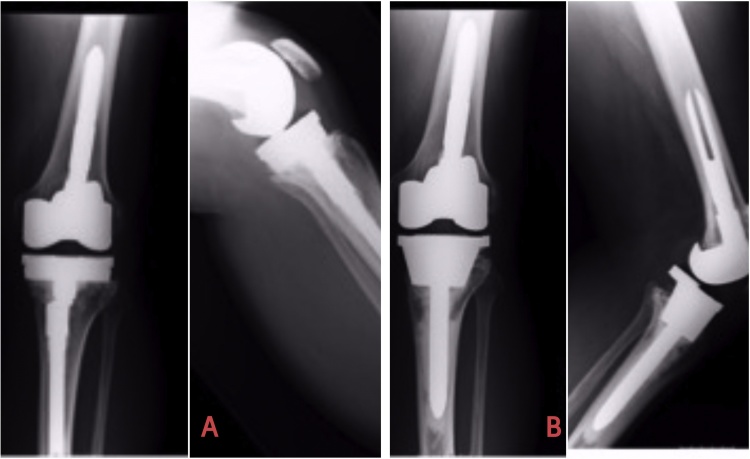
Fig. 5A shows the Genesis-2 tibial component with aseptic osteolyis and persistent pain. Note the thick metallic augment which leads to metaphyseal bone loss when explanted. This was revised to Legion tibial tray with only Tantalum cone without augment. Note the proud cone in the lateral view (Fig. 5B) and thus managing only 10 mm of Insert size (6 years F/U radiograph).
